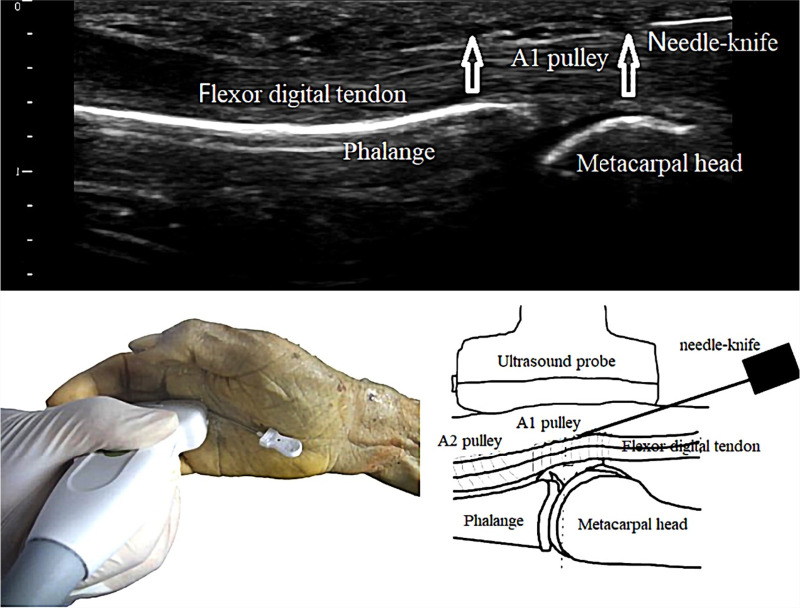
ultrasound-guided percutaneous A1 pulley release by needle-knife.
